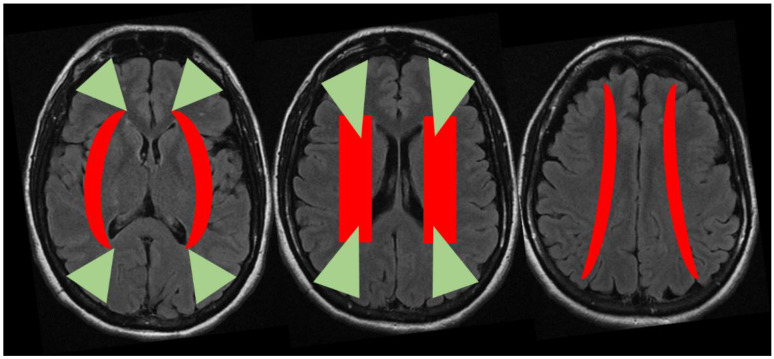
Putative location of the external (green) and internal (red) border zones superimposed on three sequential axial slices of brain MRI (FLAIR sequence). Abbreviations: FLAIR: Fluid Attenuated Inversion Recovery.
